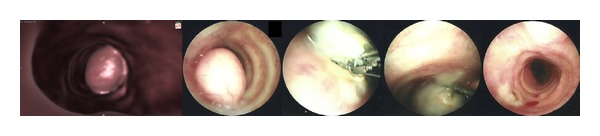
From left to right: CT reconstructed image and bronchoscopic views of the tracheal tumor and its complete resection with the use of loop electrocautery.
